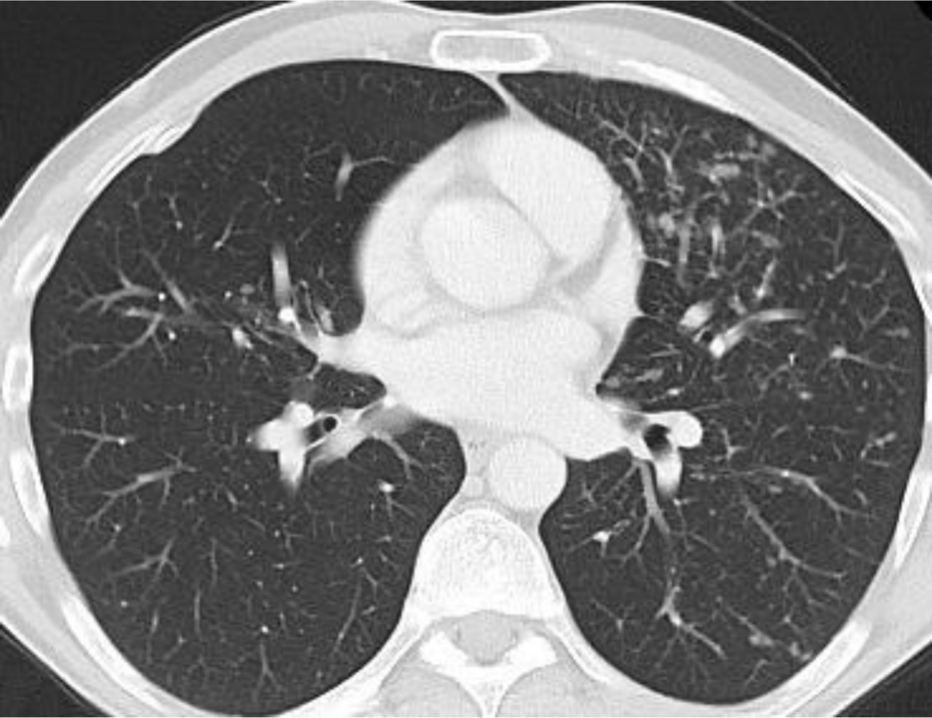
CT Thorax showing bilateral pulmonary nodular infiltrates.
